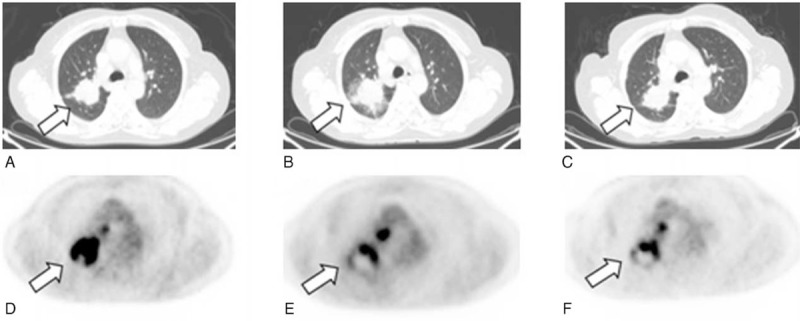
A 57-year-old woman with NSCLC (adenocarcinoma of 3.1 × 2.2 cm in size) received the PET/CT-guided RFA. Split-dose ^18^F-FDG methodology included pretreatment with 4 mCi of FDG followed by a postprocedure dose of 8 mCi for same-site treatment assessment. The size and metabolic state of the residual tumor were accurately determined in ^18^F-FDG PET/CT scans before RFA (A, D), and 45 minutes (B, E) and 3 months (C, F) after the treatment, respectively. The images revealed a focal ^18^F-FDG activity in the ablation zone at 45 minutes and 3 months after the treatment.^ 18^F-FDG = ^18^F-2-fluoro-2-deoxy-d-glucose, NSCLC = nonsmall cell lung cancer, PET/CT = positron emission tomography/computed tomography, RFA =  radiofrequency ablation.
